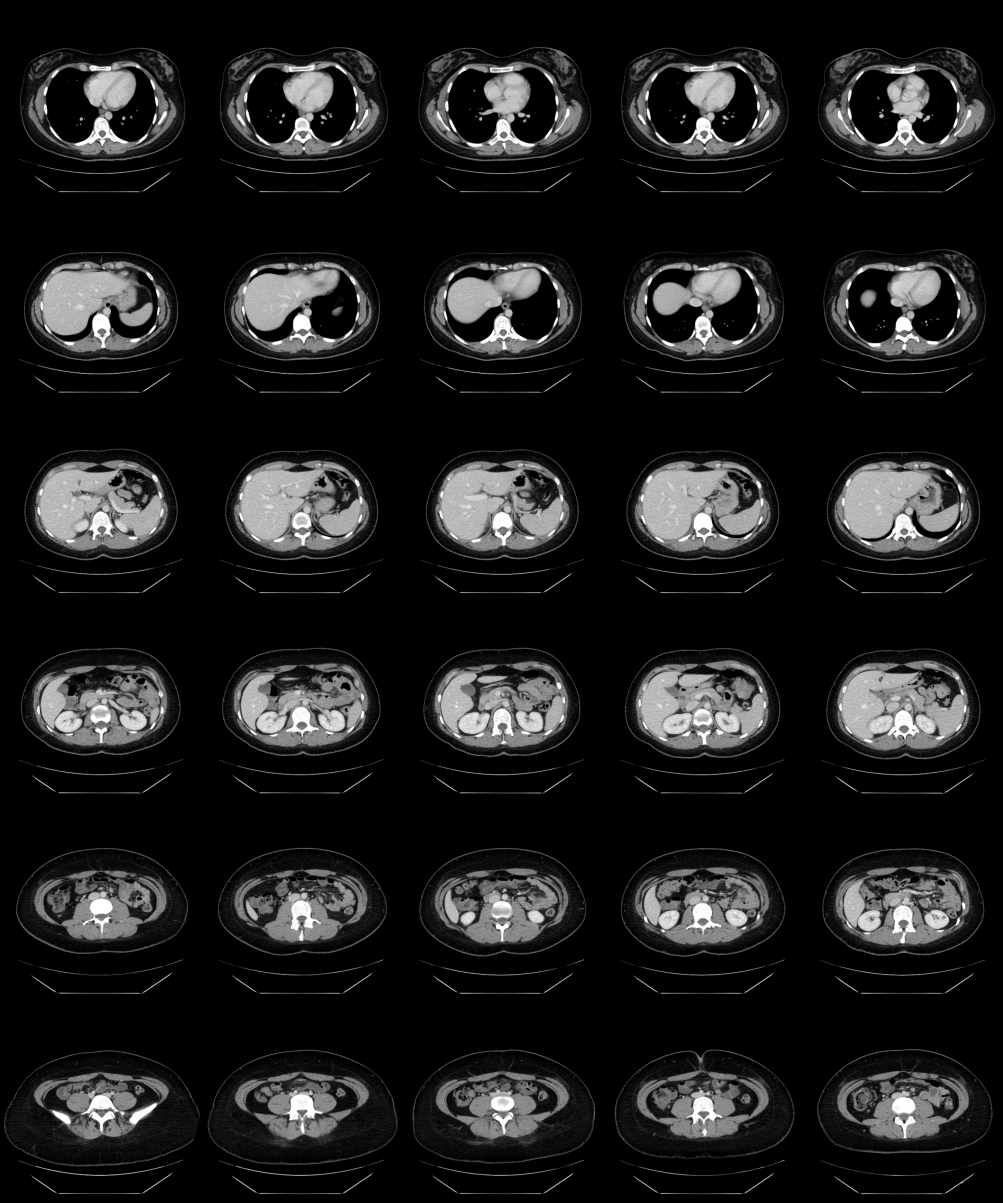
CT scan of the abdomen and pelvis showing no remarkable intra-abdominal or pelvic findings. No evidence of malignancy.
